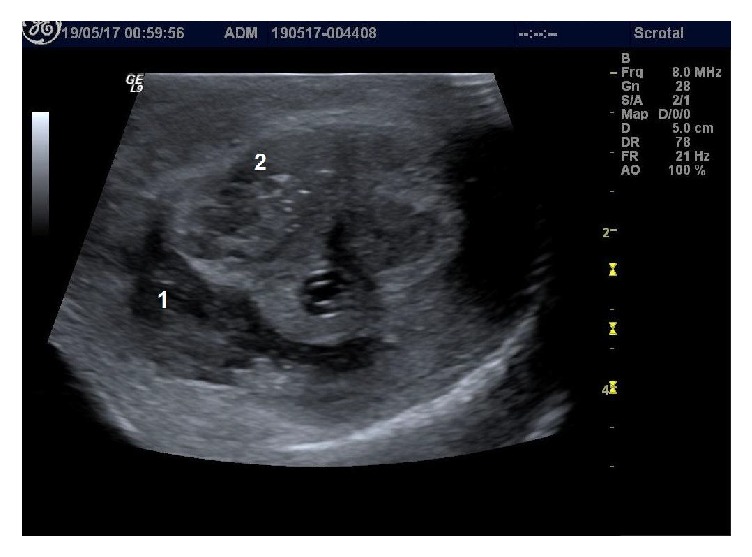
Preoperative ultrasonography of the penile shaft demonstrating hyperechogenic fluid collection with gas at the middle of the penile shaft, in contact with the right corpus cavernosum and corpus spongiosum (1) and gas in the right corpus cavernosum (2).
